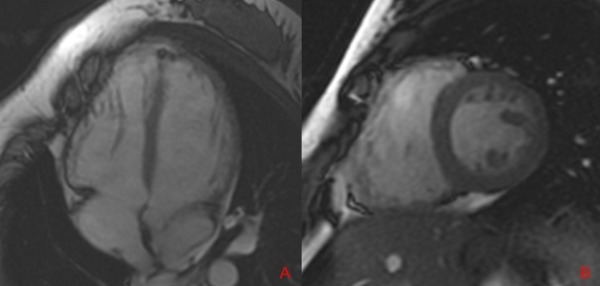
Cardiac MRI demonstrating trabeculations and deep recesses in the right and left ventricle in the four-chamber horizontal long axis view (A) and two-chamber short axis view (B).
